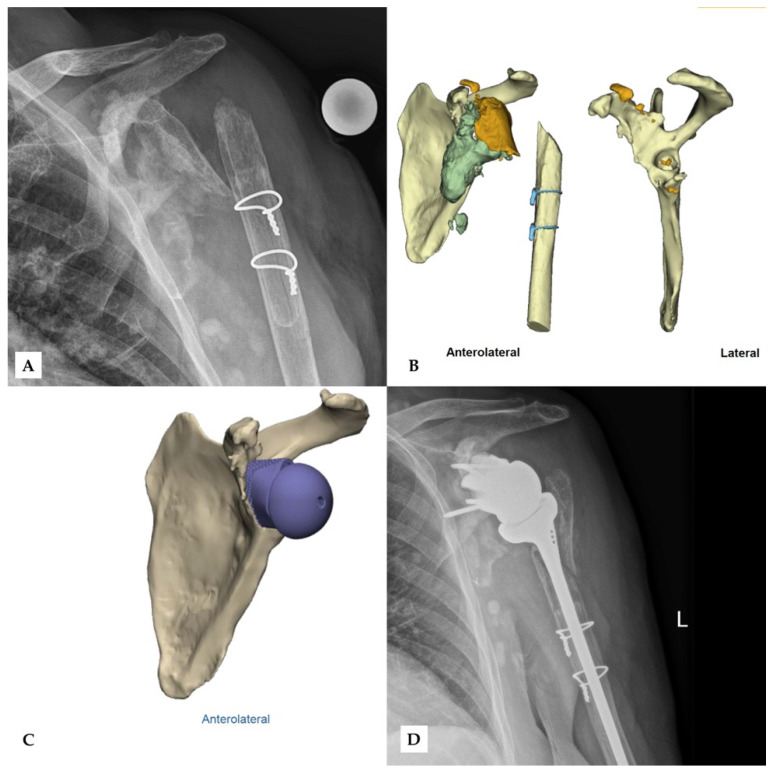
Second case of revision rTSA. The preoperative X-ray (A) after removal of the prior implant shows extensive damage of the glenoid and the proximal humerus. Bone fragments (orange) and excess cement (grey) can be seen in the CT-scan (B), while the preoperative model (C) shows the scapula after the removal of these fragments. The final outcome shows the glenoid implant in the correct position (D). Reprinted with permission from Materialise. ©2021 Materialise NV.
